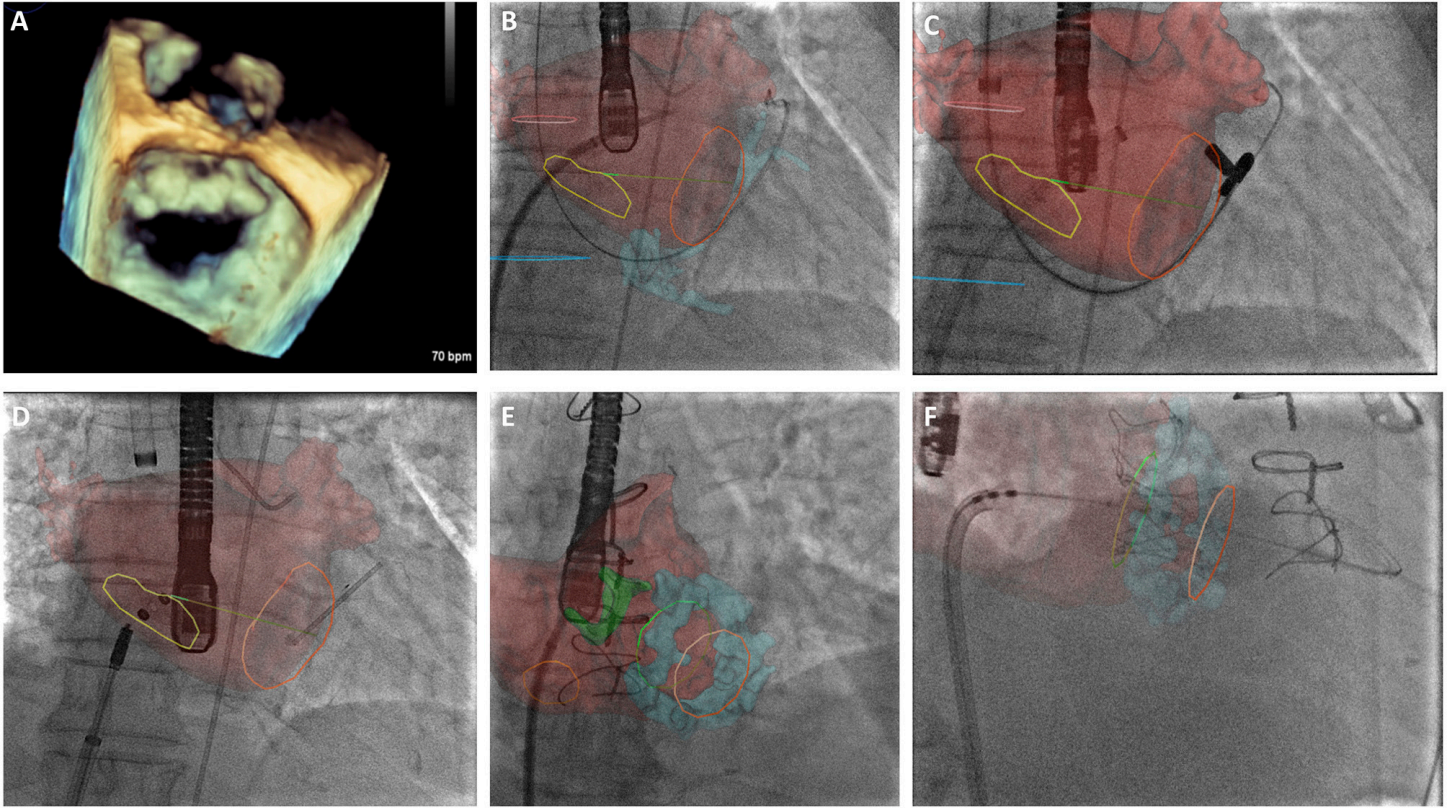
(A) 3-Dimensional transesophageal echocardiographic (TEE) real-time reconstruction of the mitral valve annulus and leaflets as a preliminary investigation to determine anatomical suitability for transcatheter mitral intervention. (B) CT overlay with real-time image fusion to demonstrate the optimal site for trans-septal puncture for ARTO case. Yellow line delineates inter-atrial septum, left atrium is superimposed in red. (C) CT overlay with real-time image fusion during magnet positioning during ARTO case (D) real-time image fusion demonstrating T-bar and atrial septal defect (ASD) occluder device device positioning relative to mitral annulus (orange circle) and inter-atrial septal markers (yellow circle), respectively. (E) CT overlay with real-time image fusion during transeptal puncture for transcatheter mitral valve implantation in mitral annular calcification identifying interatrial septum (orange circle), atrial anatomy (red) and mitral annular calcification (blue), aortic bioprothesis is also delineated (green). (F) CT overlay with real-time image fusion to facilitate transcatheter mitral valve in MAC positioning, atrial anatomy (red) and mitral annular calcification (blue) are visualized in addition to the superior (green) and inferior markers (orange).
